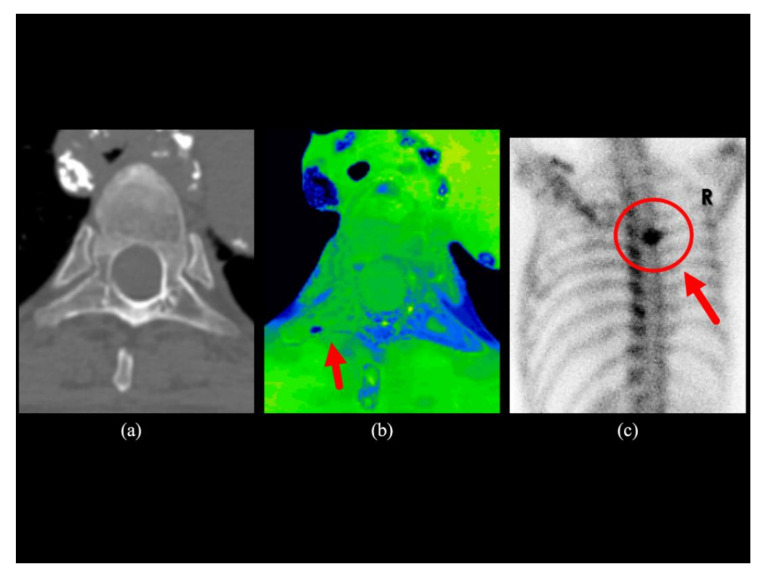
Clinical case 3. A man in his 70s with prostate cancer. (a) The transaxial image of the conventional CT image does not indicate an abnormal concentration in the fifth thoracic vertebra; (b) There is an increase in water density compared to the contralateral side in the right rib process of the 5th thoracic vertebra in the transaxial water-HAP image; (c) The posterior view of bone scintigraphy of shows metastasis to the right posterior element of the fifth thoracic vertebra. HAP; Hydroxyapatite.
